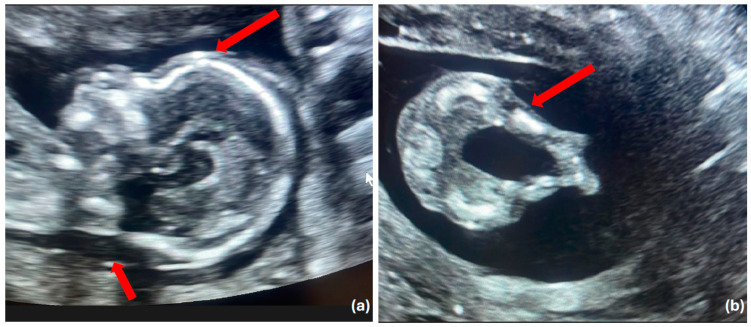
Prenatal ultrasound findings of fetus with skeletal ciliopathies. Skeletal ciliopathies represent a diverse group of disorders, including Jeune asphyxiating thoracic dystrophy (JATD), short-rib polydactyly syndrome (SRPS), Mainzer–Saldino syndrome (MZSD), and cranioectodermal dysplasia (CED). These conditions are typically characterized by a narrow chest with shortened ribs and polydactyly and often present with additional kidney, liver, and retinal diseases [1,2]. (a) Median sagittal ultrasound demonstrating increased nuchal translucency and frontal bossing. The increased NT, commonly associated with chromosomal abnormalities, when combined with the presence of frontal bossing, raises suspicion for more complex syndromic diagnoses, including ciliopathic disorders that affect both skeletal development and facial morphology (red arrows). (b) Transverse ultrasound showing short and curved femurs (red arrow). These findings are notable markers that may suggest an underlying skeletal ciliopathy [2]. A healthy 33-year-old multigravida woman was referred to our clinic at 17 weeks of gestation due to the presence of increased nuchal translucency measuring 5.1 mm. She had a history of three previous pregnancies, one of which ended in spontaneous abortion, another was terminated due to the presence of cystic hygroma, and one ended with a healthy child. She was otherwise healthy with no significant family history. Prenatal ultrasound revealed in addition to increased NT, shortened and thick long bones, hypoplastic tibia and fibula, referred absence of the bladder, a flat nose, and frontal bossing (Figure 1 and Figure 2).
